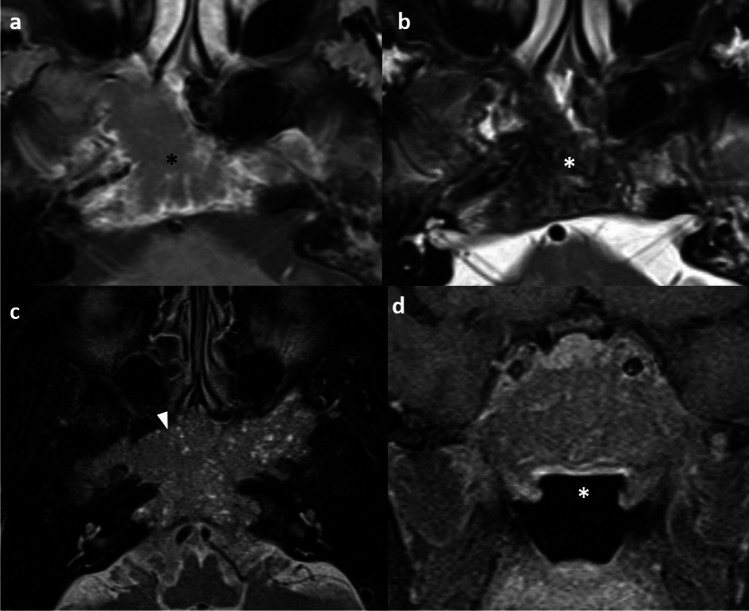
Sphenoid sinus squamous cell carcinoma in a 63-year-old man presented with headache and right 6th nerve palsy (a & b). Initial plain CT brain showed a hyperdense right sphenoid mass with clival destruction (not shown). Axial post-contrast T1-weighted image with fat-suppression shows an irregular and infiltrative mass centered at right sphenoid sinus with clival, bilateral petrous apex destruction, and intracranial extension (not shown). The lesion shows irregular peripheral enhancement with central areas of hypo-enhancement (asterisk) (a). Axial T2-weighted image with fat-suppression shows the corresponding mass with heterogenous, predominantly hypointense signal (asterisk) (b). Invasive pituitary macroadenoma in a 73-year-old woman presented with persistent headache (c & d). Axial T2-weighted image with fat-suppression shows an extensive tumor mass with intermediate signal containing multiple internal cystic foci involving the central skull base (arrowhead) (c). Coronal post-contrast T1-weighted image with fat-suppression shows the lesion centered at the sella turcica and clivus with bilateral cavernous sinus extension. The nasopharyngeal roof is unremarkable (asterisk) (d)
